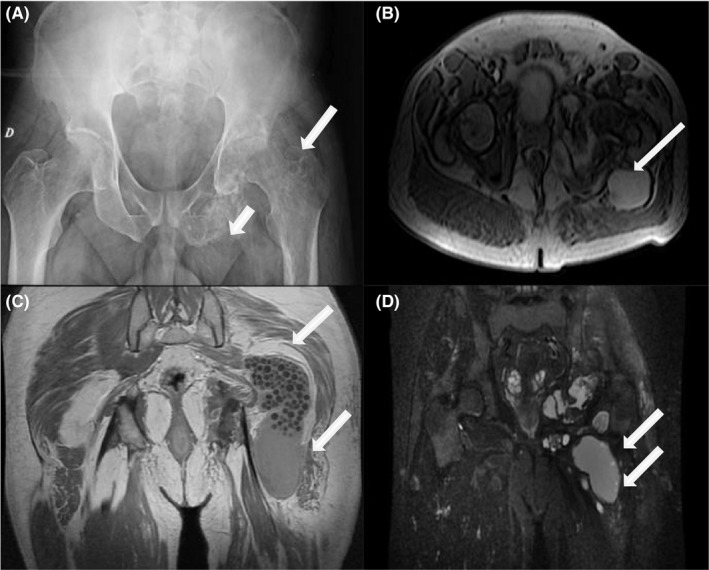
(A) Pelvic X‐ray showing osteolytic lesions in the ischiopubic branch and all the left femur and proximal extremity of the tibia. (B) MRI of the pelvis: axial section in T1 gado sequence sowing multilocular appearance of the femoral head with breach of the bone cortex of the femur with a very large intra‐articular effusion. (C) Coronal section in T1 gado sequence with Fat Sat sowing involvement of the ischial branch of the pubis and a very large intra‐articular effusion and multilocular appearance of the soft parts. (D) Coronal section in T1 sequence sowing multilocular appearance of the soft parts
